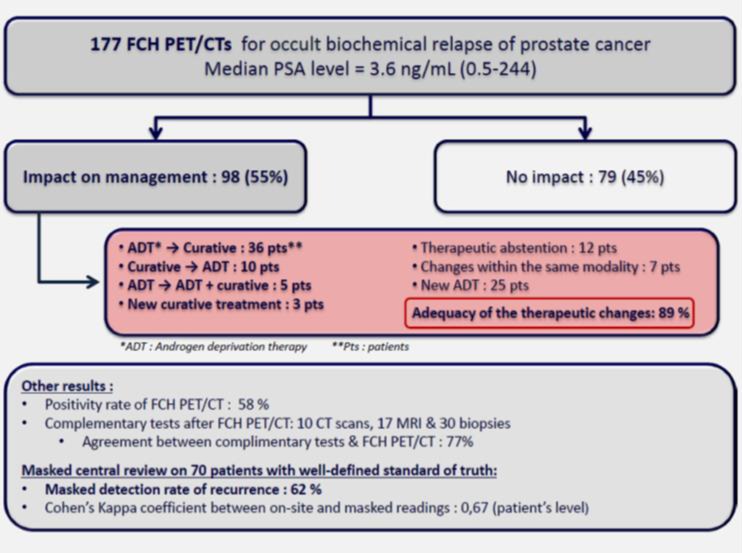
Gillenbert et al. [1] showed in a large prospective multicenter clinical trial comprising 177 patients with biochemical relapse from prostate cancer that ^18^F-Choline-PET/CT has a major impact on the therapeutic decision making process in 55 % of patients. An expert panel rated almost 90 % of therapeutic changes as adequate. However, a positivity rate of 58 % indicates that a significant proportion of patients has negative imaging results, and thus do not benefit from PET/CT imaging
